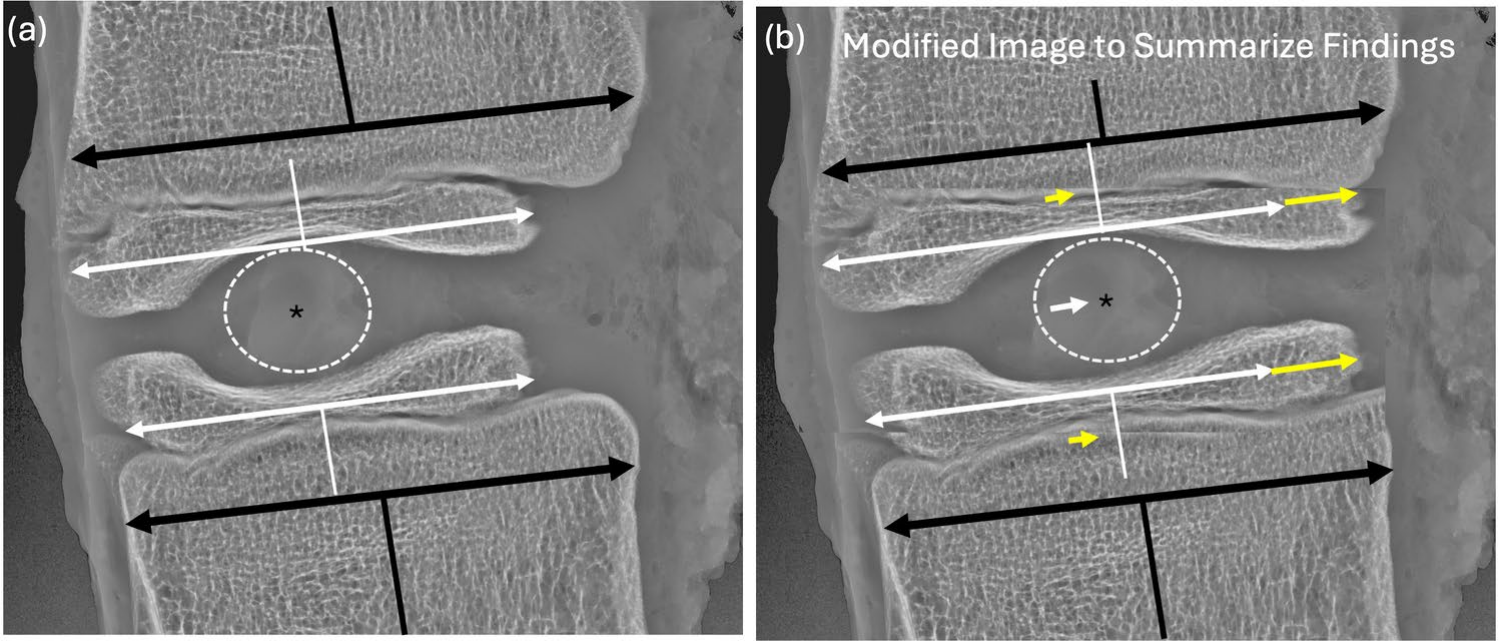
Modified radiographic images to summarize the interactions between NP and epiphyseal ossification. In the kyphotic spine the center of the ossified epiphysis (white vertical lines and double arrows) is positioned posterior to the center of the vertebral body (Black vertical lines and double arrows) while the center of the NP (asterisk) sits posterior to posterior to the center of the ossified epiphysis and therefore, very posterior to the center of the vertebral body. Tethering appears to shift the center of the NP toward the center of the ossified epiphysis (small single white arrow), while this is occurring the anterior epiphysis is also ossifying (yellow arrows), thus the NP is also shifting toward the center of the vertebral body. This suggests that in addition to correcting spinal deformity by the altering vertebral body shape through growth modulation at the physis, structural changes are also occurring in the epiphysis following pVBT
